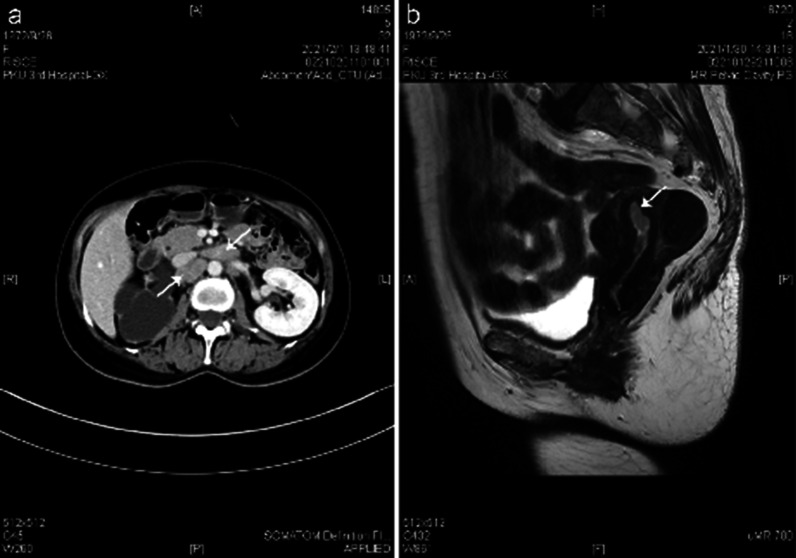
Imaging findings of CTU and MRI. a The arrows pointed to the enlarged lymph nodes. b The arrow pointed to thickened endometrium
